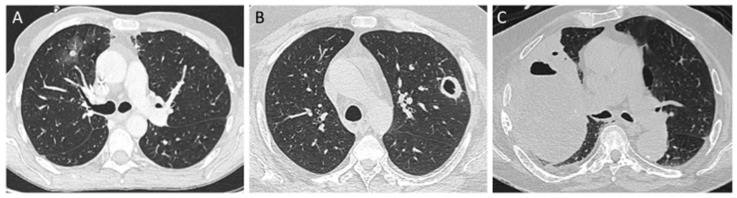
Different CT scan findings in pulmonary mucormycosis. (A): Axial pulmonary CT scan shows a nodule surrounded by ground-glass opacity in a patient with hematological malignancy. (B): Axial CT scan reveals excavated pulmonary nodule in a diabetic patient. (C): Axial CT scan shows voluminous mass with costal extension in a patient with lung cancer.
